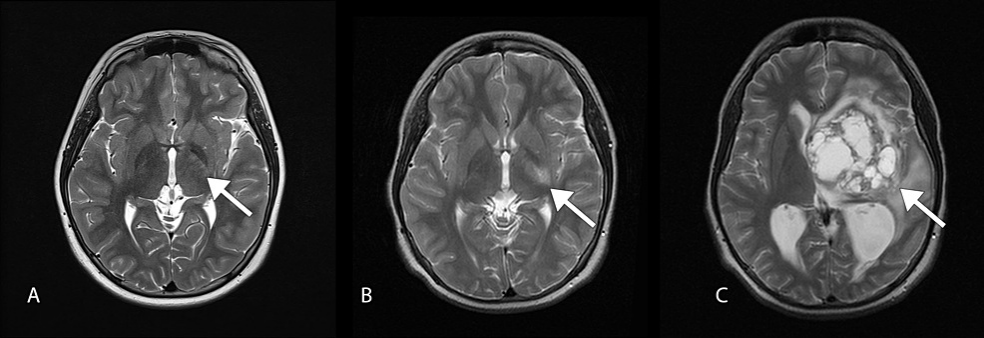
Axial T2-weighted image showing the temporal evolution of the left basal ganglia germinoma. Initial MRI in 2017 showing minimally hyperintense foci in the left basal ganglia (A, white arrow). In 2018, small cystic foci were noted in the same region of the left basal ganglia (B, white arrow). The latest MRI in 2020 showing progression into a large mass with multicystic appearance and midline shift to the right leading to obstructive hydrocephalus (C, white arrow). The mass is surrounded by perilesional white matter edema.
